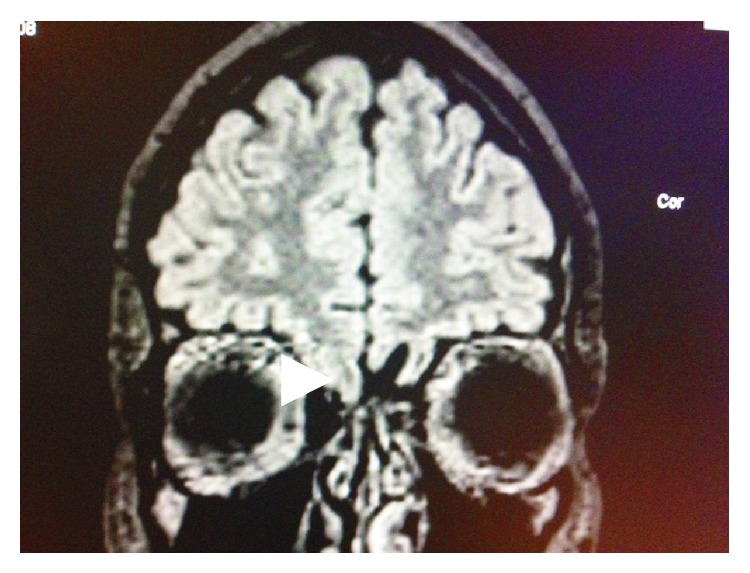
MRI for patient with skull base defect and possible CSF leakage. Gyrus rectus protrusion.
